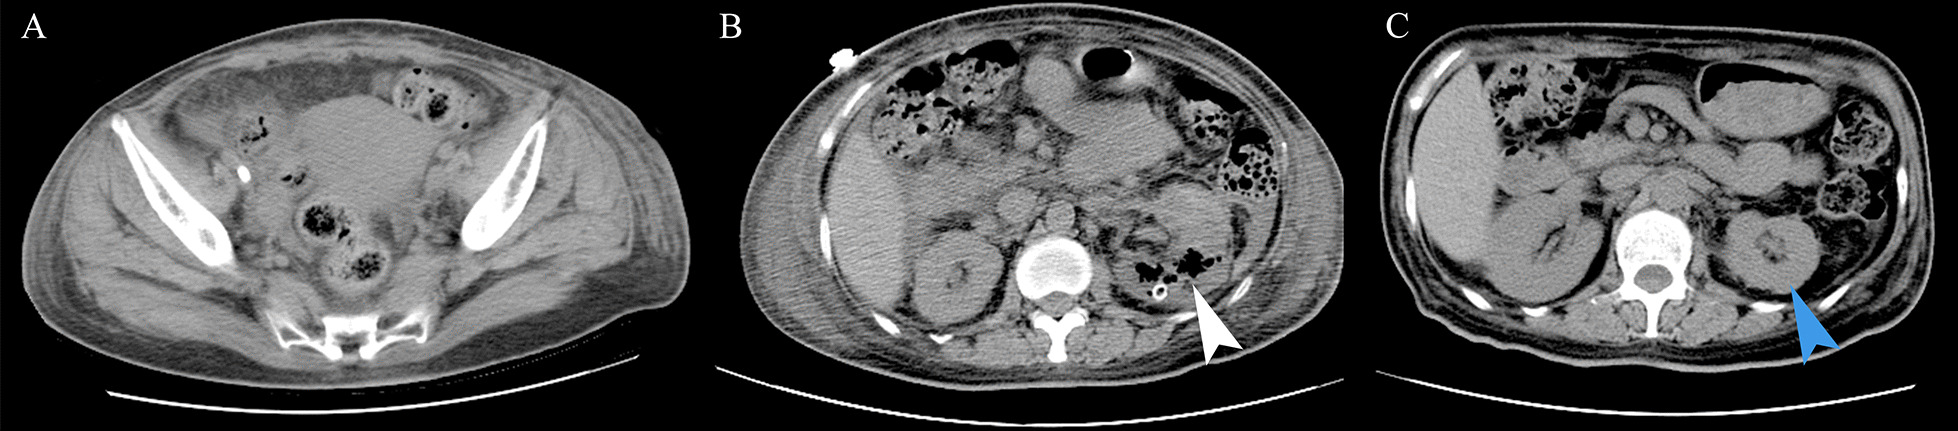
The follow-up computed tomography scan. A, B Abdominal and pelvic CT taken 3 days after open drainage show reducing of gas the uterine vein and in the left kidney (white arrow). C The 3-month follow-up abdominal CT shows complete absence of gas and shrunk-volume in the left kidney (blue arrow)
